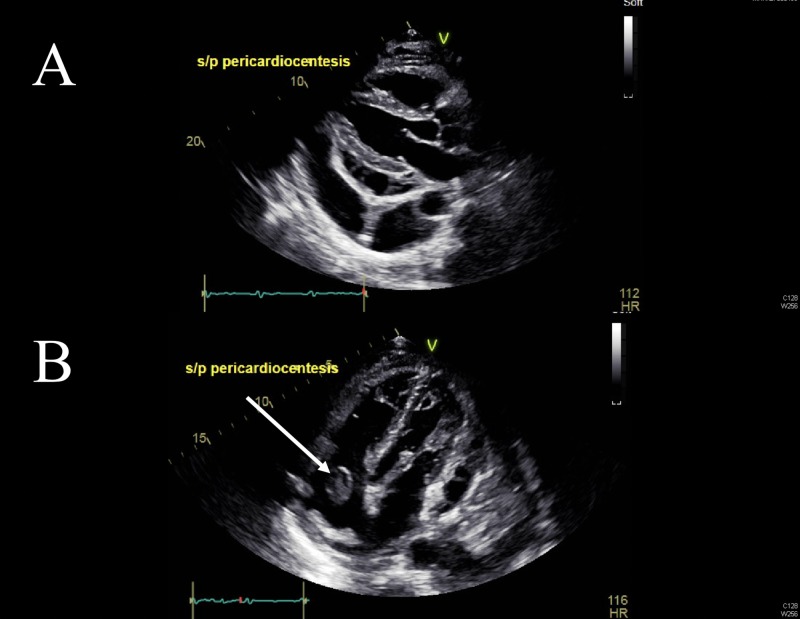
Presenting Transthoracic Echocardiographic Views A) Long axis and B) apical four chamber echocardiographic views at presentation showing the pericardial effusion and evidence of the thrombus in transition through the right atrium. S/P: status-post
